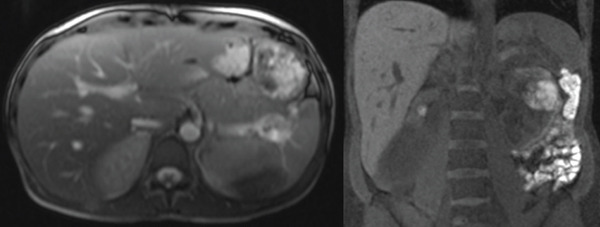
MRI showing an exophytic 6.2 × 4.2 cm lesion in the interpolar region of the left kidney later determined to be RCC. Additionally, multiple T2 hyperintense liver lesions, measuring up to 1.7 cm, can be appreciated.
